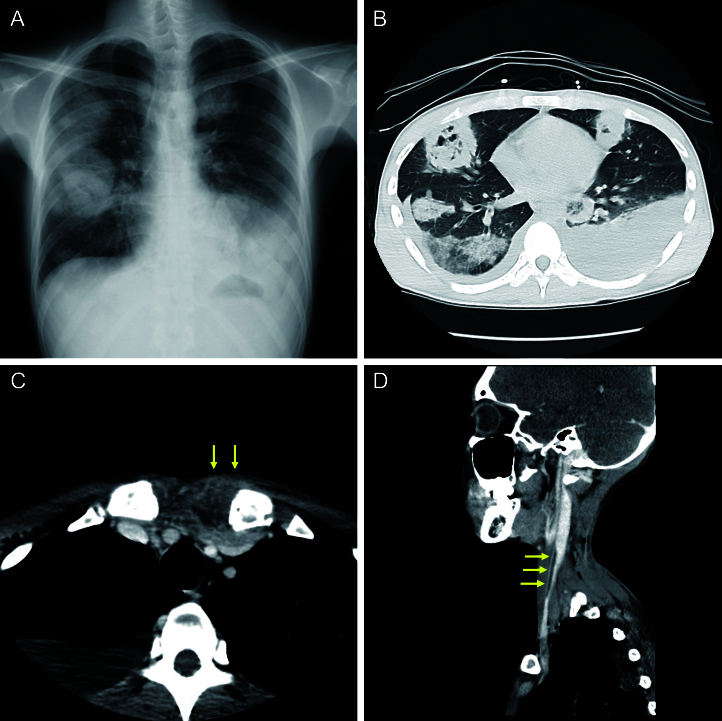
(A) Chest radiograph revealing multiple large, rounded opacities in both lung fields. (B) Chest computed tomography (CT) revealing multiple bilateral pulmonary cavities and bilateral pleural effusions. (C) Contrast-enhanced CT revealing increased thickness of the soft tissue around the left sternoclavicular joint (arrows) and (D) a filling defect in the right internal jugular vein (arrows).
